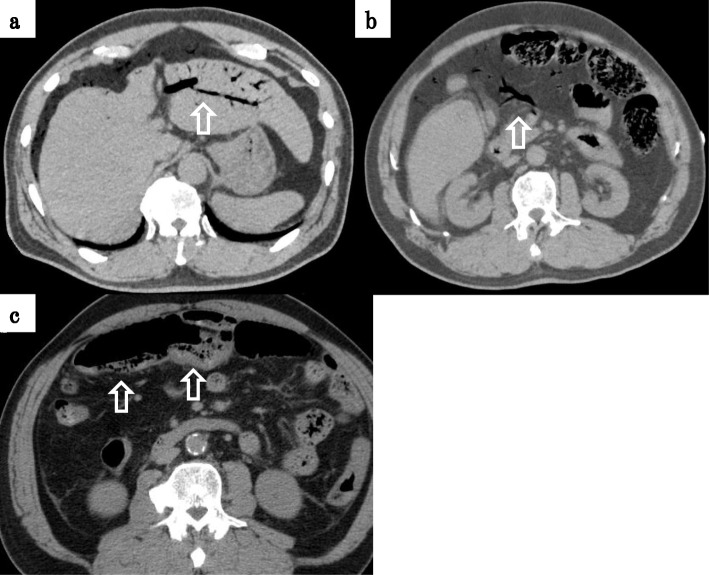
CT imaging before HBOT. a A large amount of intravenous gas was detected in the portal vein (white arrow) and b superior mesenteric vein (white arrow). c CT imaging after transfer to our hospital revealed pneumatosis intestinalis of the transverse colon (white arrow)
